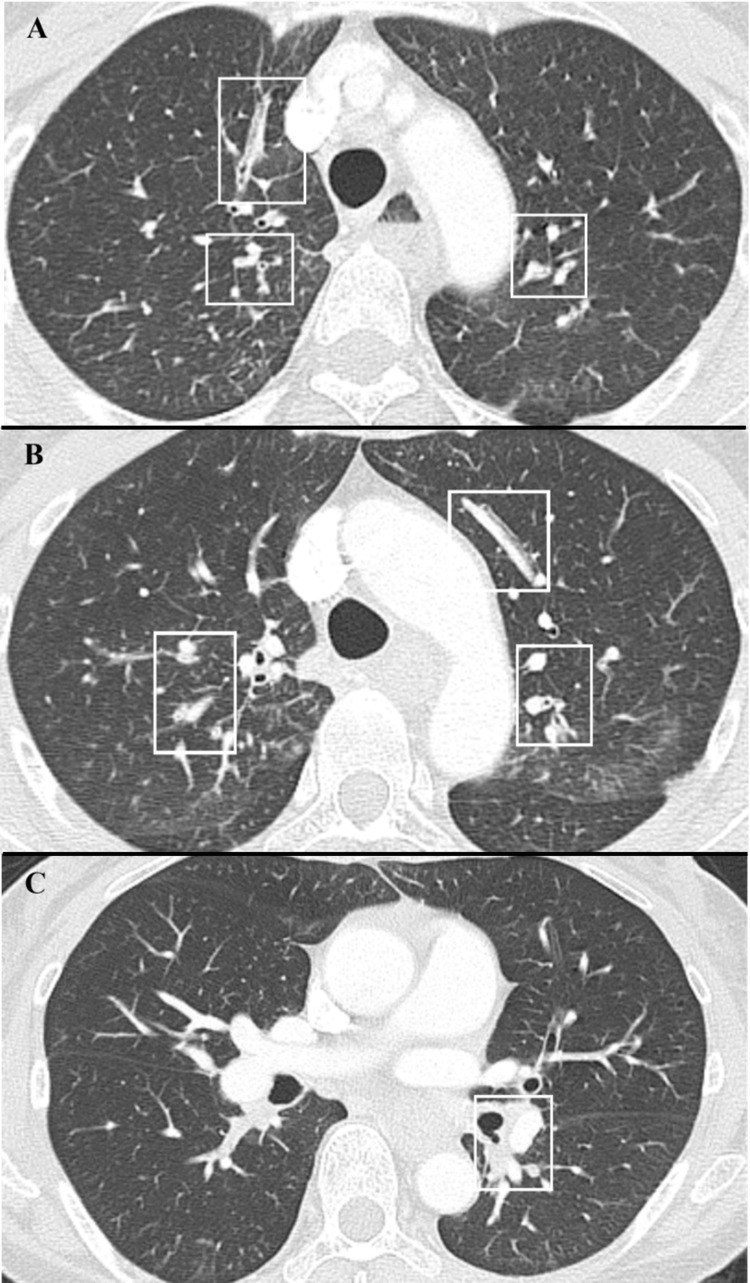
Computed tomography image of the chest Chest CT showed peripheral bronchial wall thickening, lumen narrowing, and obstruction in the bilateral upper lobes and left lower lobe (A, B, and C) (□).
